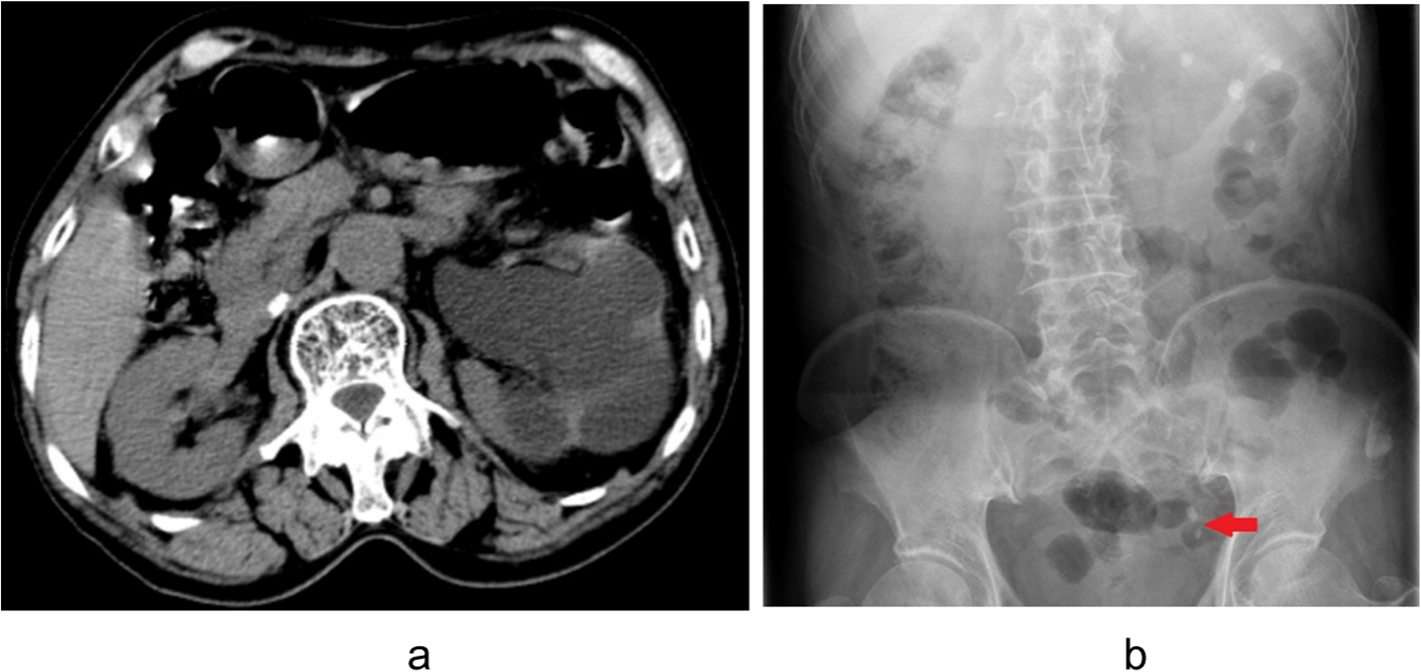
a CT revealed mild hydronephrosis in the left kidney. b KUB exhibited two lower ureteral stones
